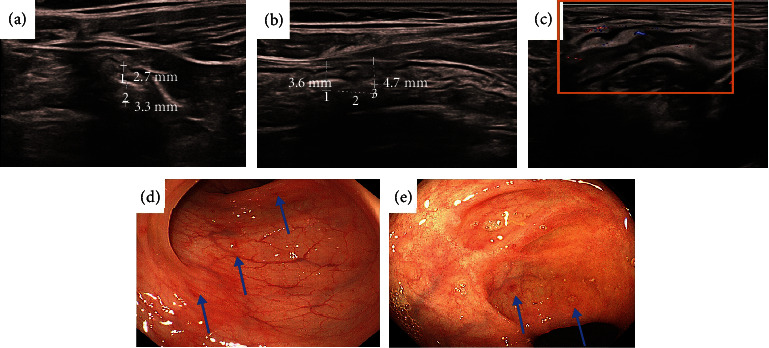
This female CD patient was 20 years old, and the patient had clinically inactive CD (CDAI = 41), which was characterized by diarrhea. In our examinations, this was a non-moderate-severe CD. The SES-CD and IBUS-SAS were 3 and 18.3 points, respectively. The serious segmental was the sigmoid colon. Application of the segmental activity and severity scores. (a, b) Bowel wall thickness (BWT): In longitudinal and cross-section orientations, BWT measurements were performed, and two measurement values were given in each orientation, [BWT] = [2.7 + 3.3 + 3.6 + 4.7]/4 = 3.58 mm. (c) Blood flow/color Doppler signal [CDS] = 1[short signals]. Bowel wall stratification[BWS] = 0, inflammatory fat[I‐fat] = 0 (d), and (e) endoscopic image showing several aphthaes (arrow). IBUS‐SAS = 3.58 × 4 + 0 × 15 + 0 × 7 + 1 × 4 = 18.3.
